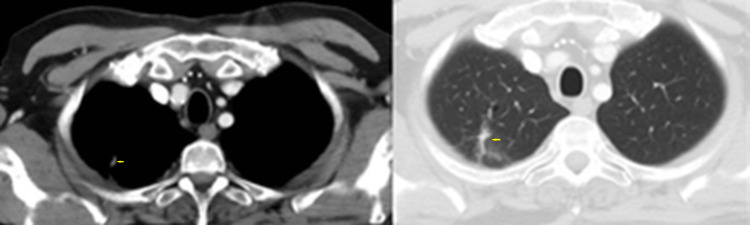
Chest computed tomography scan on June 13, 2018. Stable findings of the lungs.
